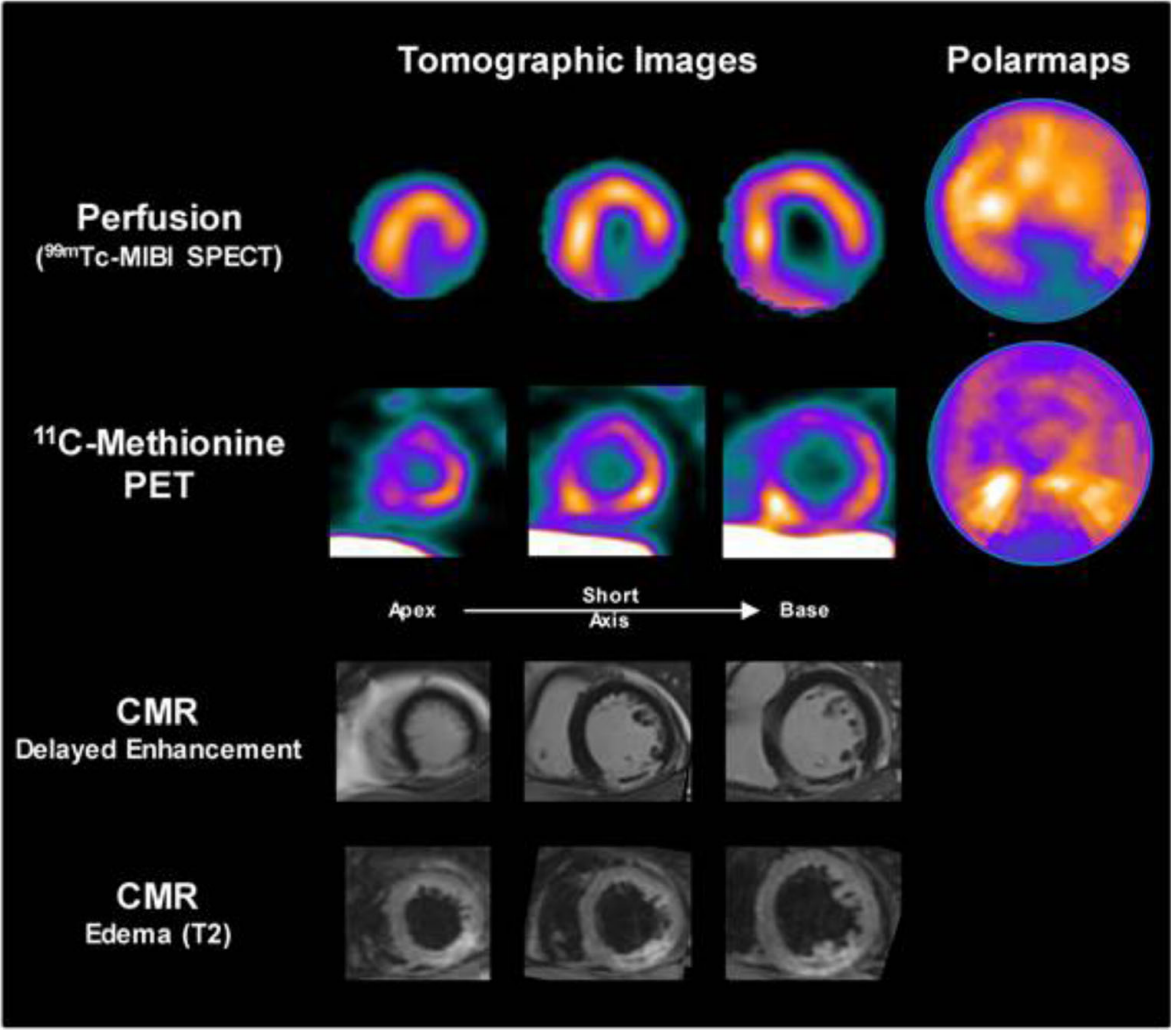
Images from a patient acquired 3 days after acute myocardial infarction and reperfusion. Perfusion SPECT and ^11^C-methionine PET cardiac images and associated polar maps display diffuse myocardial uptake with elevated ^11^C-methionine accumulation in the border zone of the perfusion defect. Cardiac magnetic resonance images confirm delayed gadolinium enhancement in the infarct region, with central no-reflow area, alongside with edema in the infarct region on T2-weighted images
